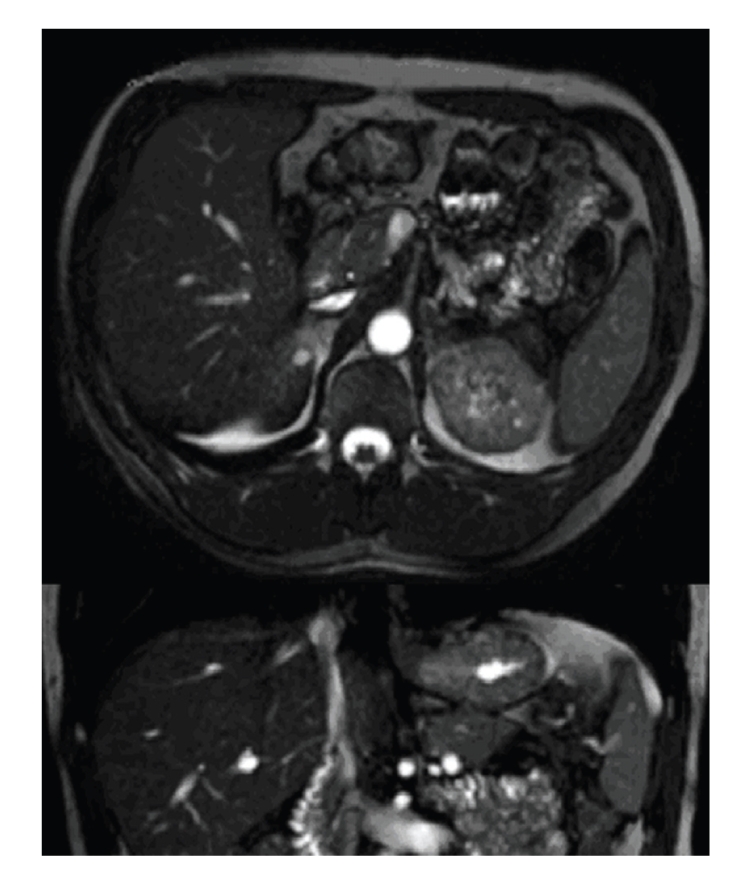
Transverse T2-weighted TSE sequences (up) and Coronal BALANCE sequences (down) do not visualize the gallbladder in the cholecystic fossa.
